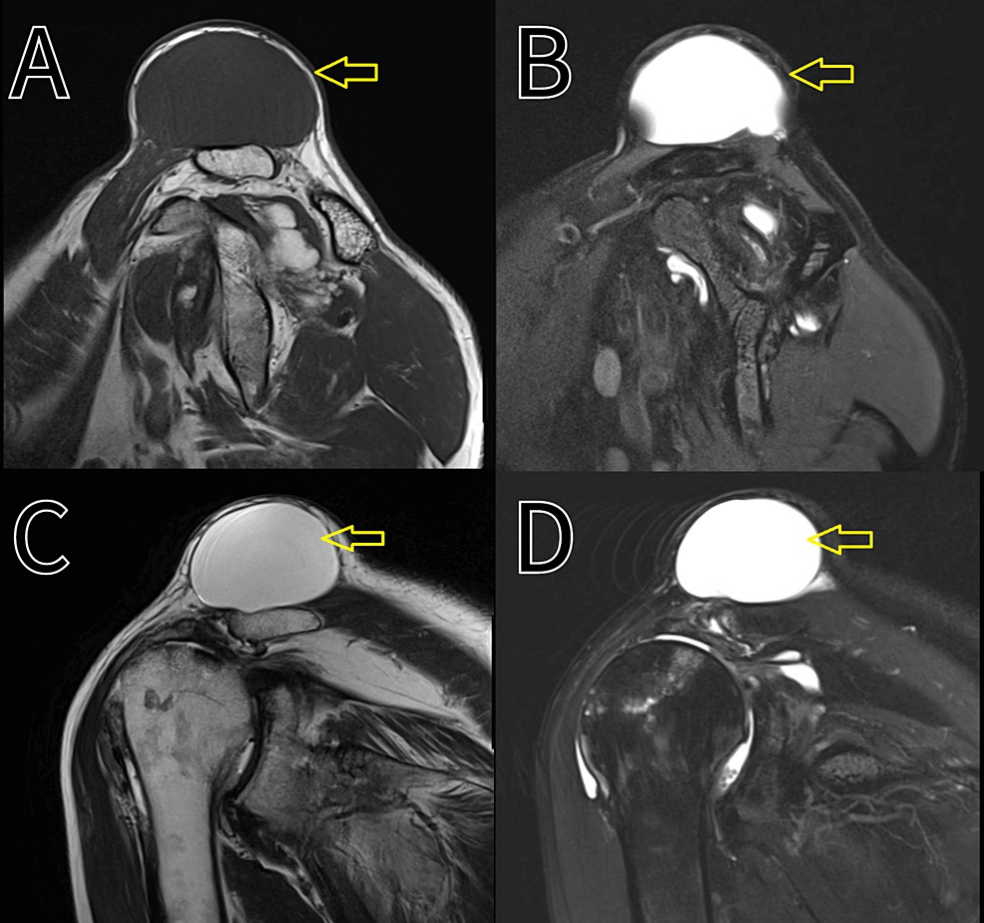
MRI showing a localised soft tissue swelling A: T1-weighted image, B: T2-weighted image, C: T2-weighted image, D: proton density fat-saturated (PDfs) image
